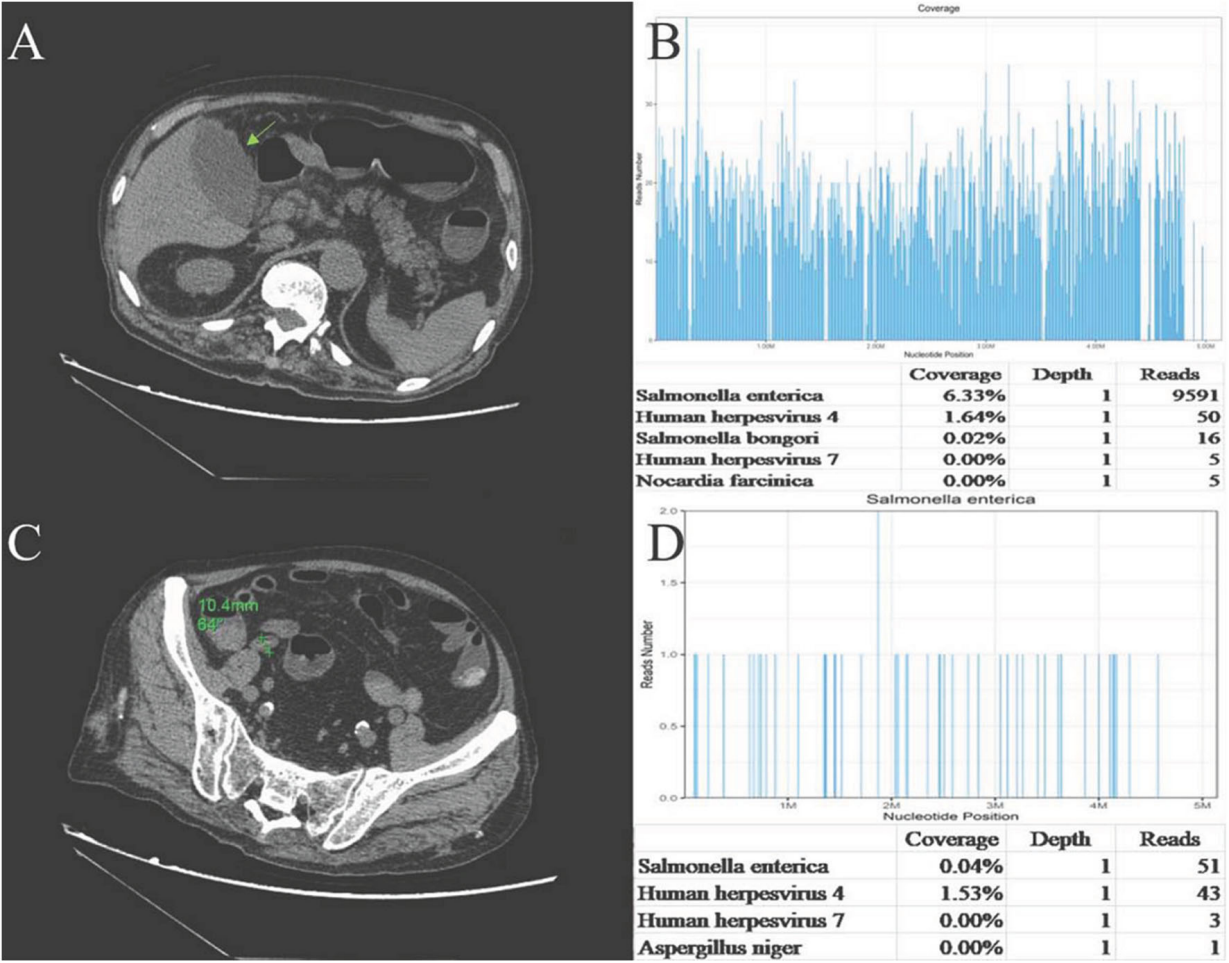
(A)Abdominal CT plain: the gallbladder enlarged, and gallbladder wall was thickened and blurred. (B) Abdominal CT plain: The appendix cavity thickened, and the widest diameter was ~11 mm. (C) Sequencing of *Salmonella* yielded a total coverage of 6.33% in the gallbladder tissue by NGS. Number of unique reads, coverage, and depth of the identified pathogen sequences. The obtained sequence information was compared and analyzed with the known microbial database of NCBI gene bank to determine the type and content of microorganisms in the sample. The more segments detected, the more coverage, and the more reliable the result. (D) Sequencing of *Salmonella* yielded a total coverage of 0.04% in the appendix tissue by NGS. Number of unique reads, coverage, and depth of the identified pathogen sequences.
